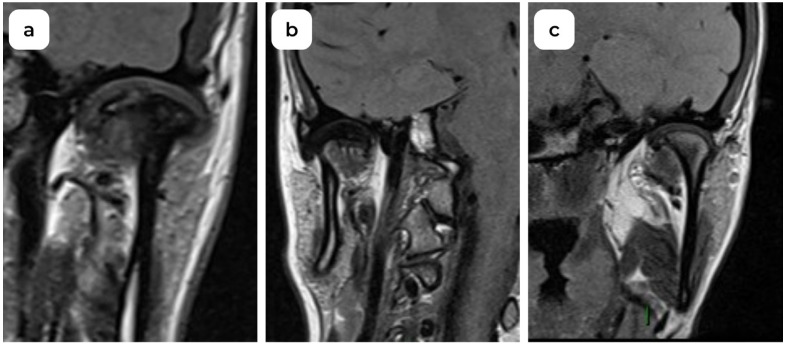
MRI oblique coronal view of TMJ: (a) normal disc position; (b) lateral disc displacement; (c) medial disc displacement.
